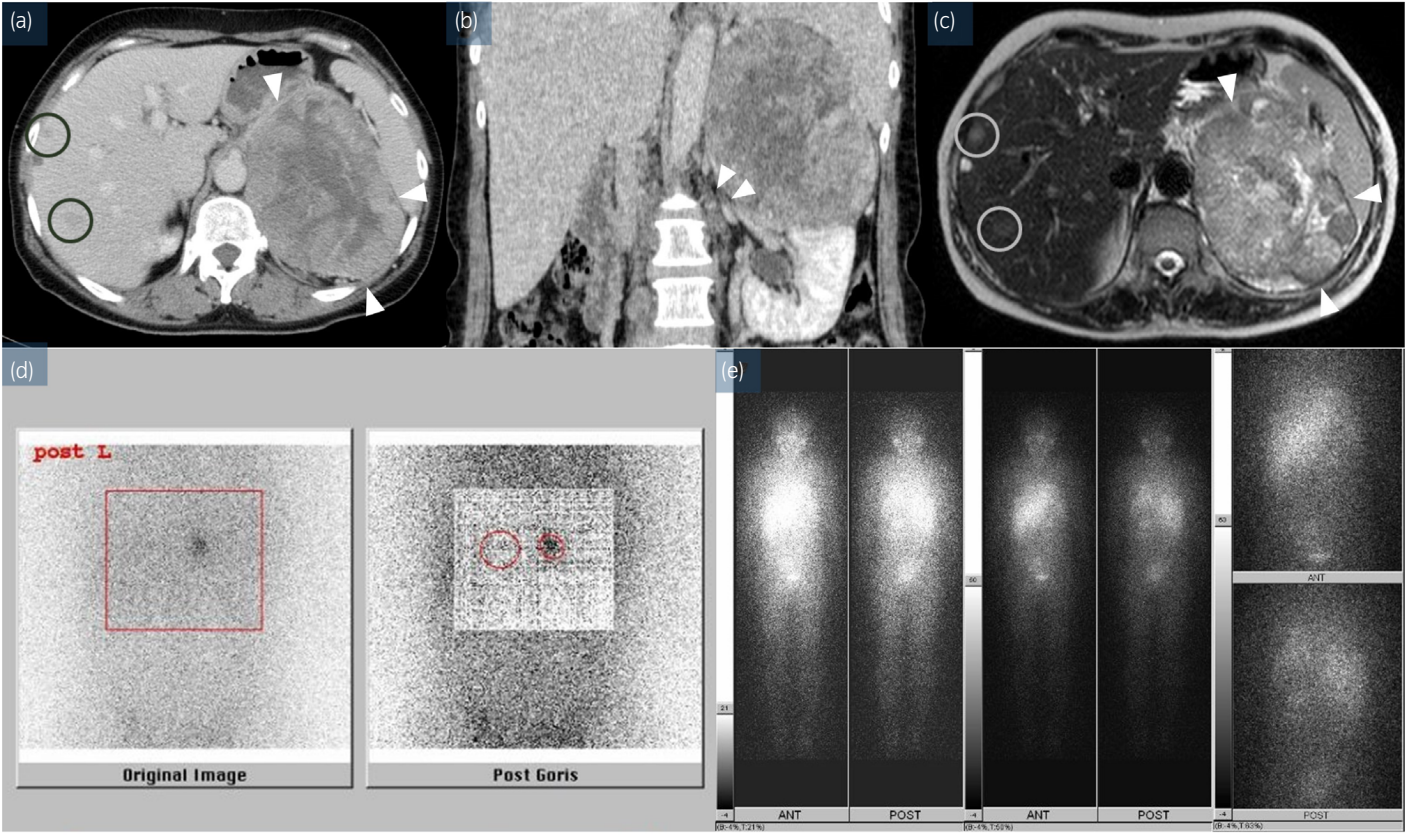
Imaging findings. Enhanced CT showing (a) a retroperitoneal tumor (arrowhead, 95 × 123 × 132 mm) and metastatic liver tumors (circle), and (b) lymphadenopathy of the hilar region of the kidney (arrowhead). (c) Magnetic resonance imaging (T2WI) showing a retroperitoneal tumor (arrowhead) and metastatic liver tumors (circle). (d) ^131^I‐adsterol scintigraphy showing no signal accumulation in the left adrenal gland (circle). (e) ^131^I‐MIBG scintigraphy showing no signal accumulation in the retroperitoneal tumor. [Colour figure can be viewed at wileyonlinelibrary.com]
